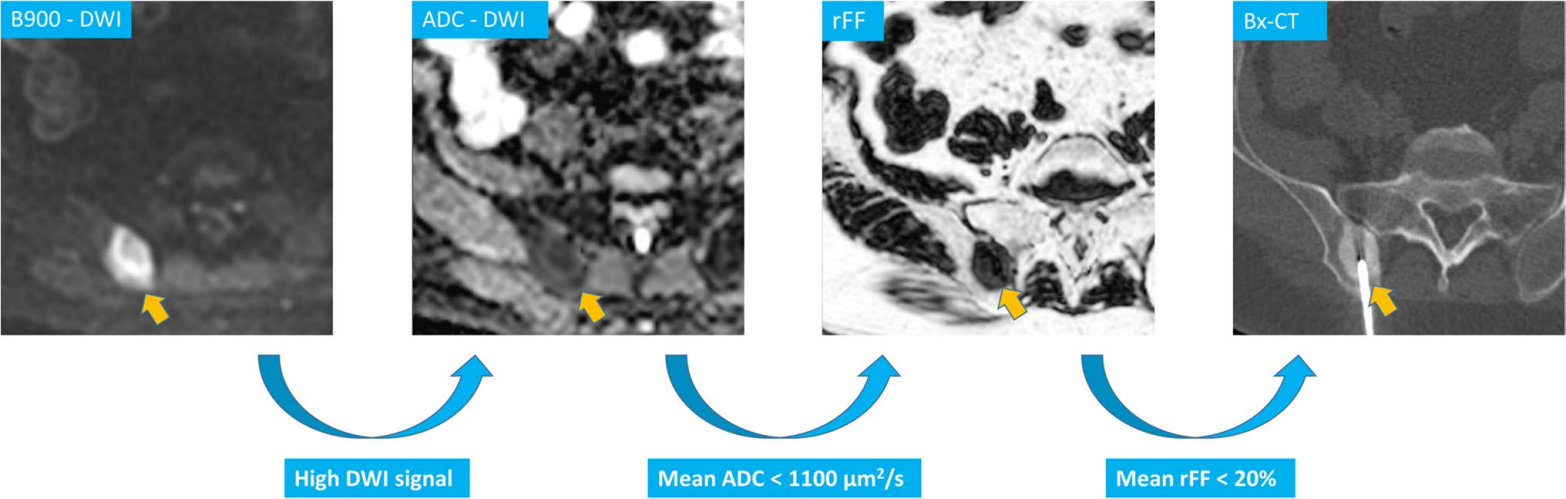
Right iliac crest metastasis in a 56-year-old prostate cancer patient and multiparametric MRI bone biopsy target selection algorithm, high signal DWI b900 diffusion-weighted images (DWI), low apparent diffusion coefficient (ADC), relative fat fraction (rFF), and consecutive biopsy CT (Bx-CT). Note that despite the sclerotic CT appearance, the biopsy samples were suitable for next-generation genomic sequencing
